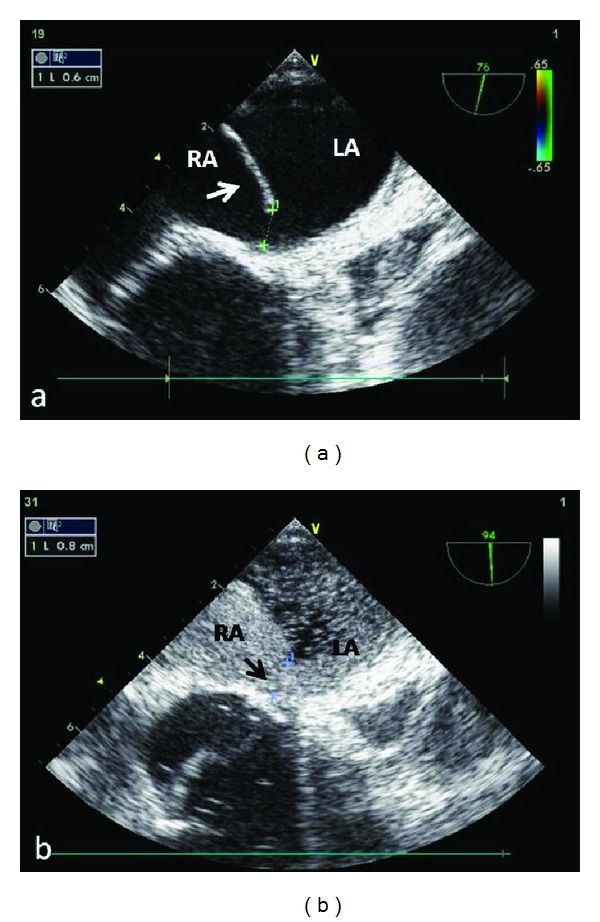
Transesophageal echocardiogram demonstrating: (a) the aneurysm of the atrial septum (white arrow) and (b) the size of patent foramen ovale (0.8 cm) with right-to-left shunting (black arrow) after agitated saline contrast injection. RA, right atrium; LA, left atrium.
